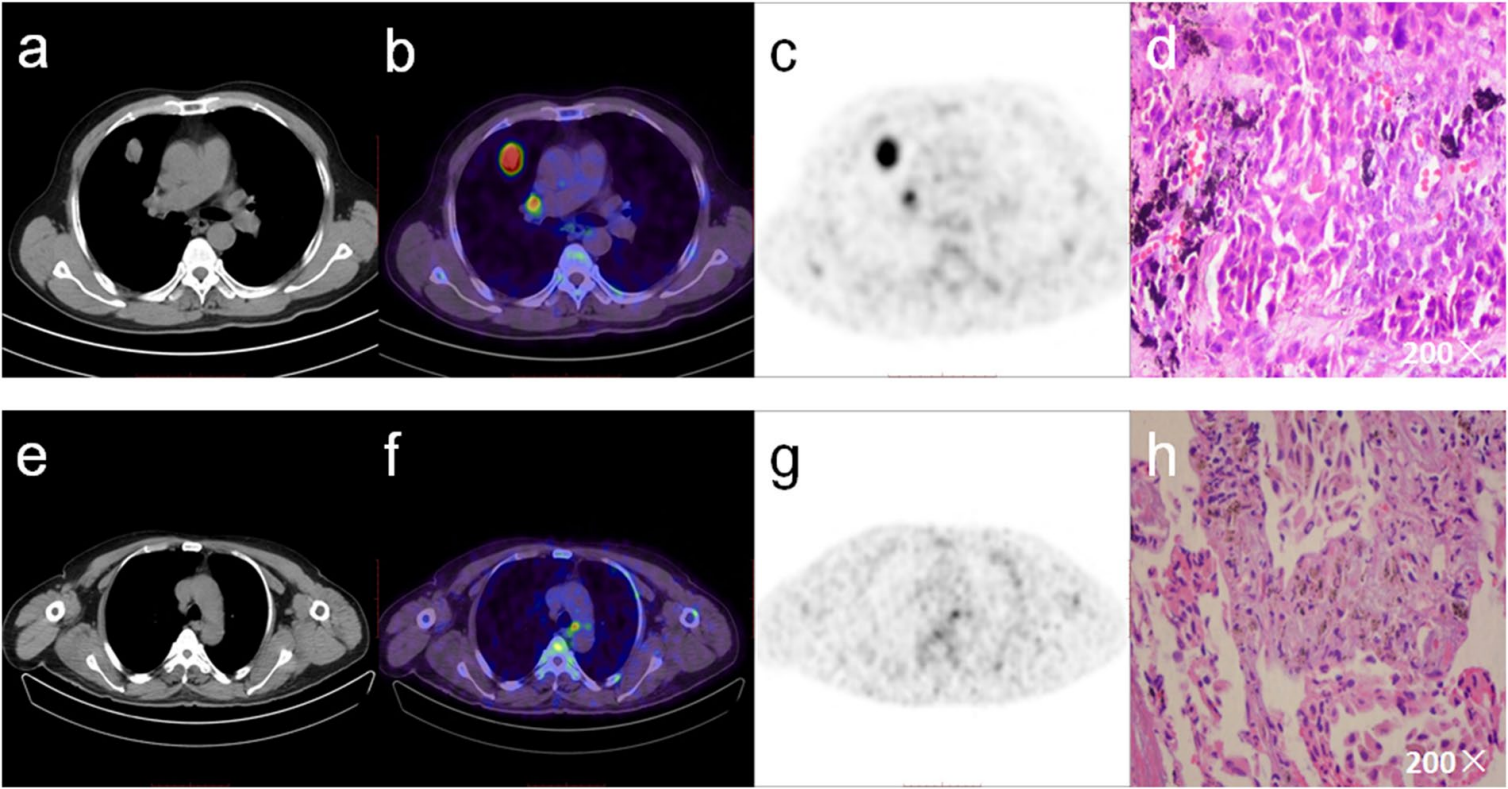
The upper row shows a 62-year-old male suffering from an adenocarcinoma of the upper right lobe. LN station 10 according to Mountain and Dresler^1^ was classified as a true positive case (a,b,c,d). The lower row shows a 59-year-old male suffering from a squamous cell carcinoma of the lower left lobe. The increased focal ^18^F-Alfatide uptake in PET/CT imaging is an example of false positive lymph node metastasis (e,f,g,h).
